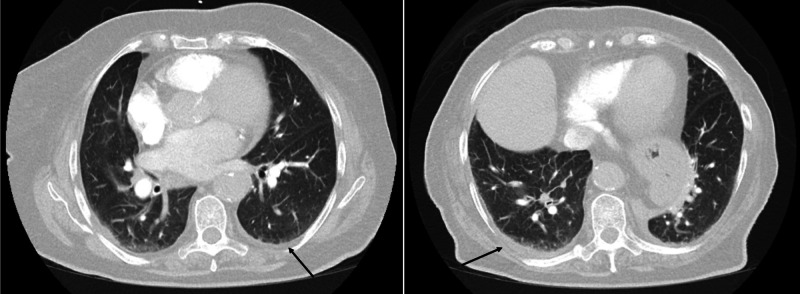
A & B. Different sections of CT of the chest obtained on admission. A & B. Black arrows showing bilateral basal atelectasis and small pleural effusions.
